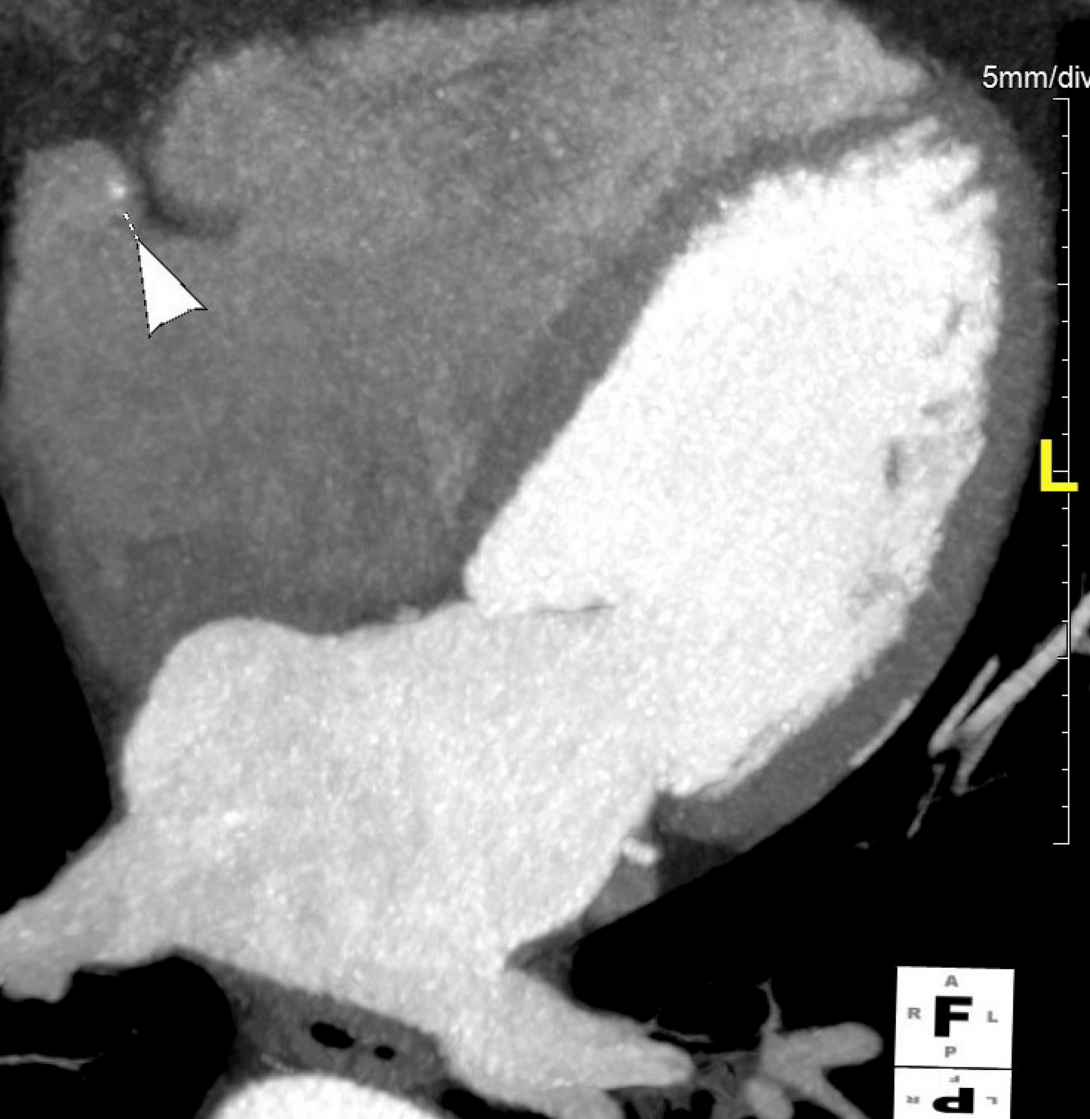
Axial MDCTA image Axial multi-detector computed tomography angiography (MDCTA) image demonstrating the intra-atrial segment of the right coronary artery (RCA), indicated by the arrowhead. The vessel is seen coursing within the right atrium, fully surrounded by intracavitary blood, confirming the anomaly.
